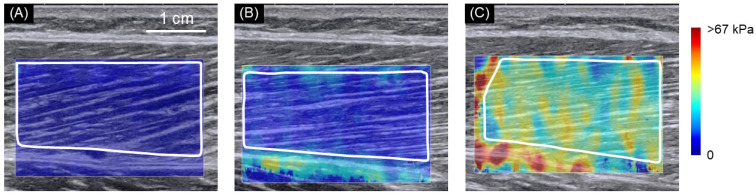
Typical examples of ultrasound shear wave elastographic (SWE) images. Typical examples of ultrasound SWE images with the knee fully extended in the supine position (A), the knee flexed to 90° in the seated position (B), and the knee fully flexed in the seated position (C). The colored region represents the shear wave speed map, with the scale to the right of the figure. The area drawn in solid white lines is the region of interest for the determination of shear modulus.
